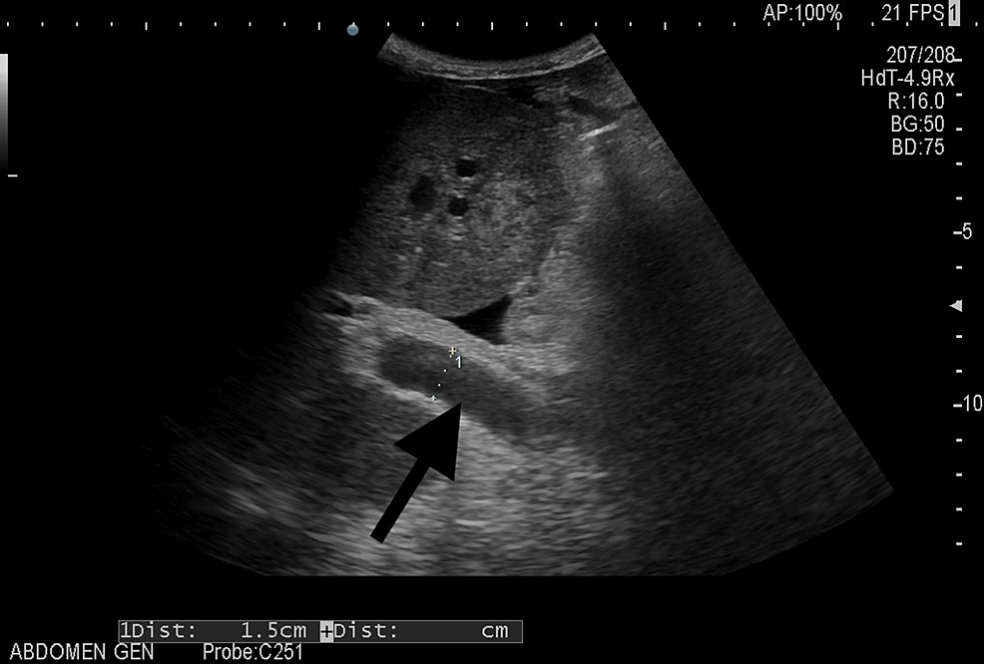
Ultrasonography of the abdomen showing a dilated portal vein measuring 1.5 cm, suggestive of portal hypertension
